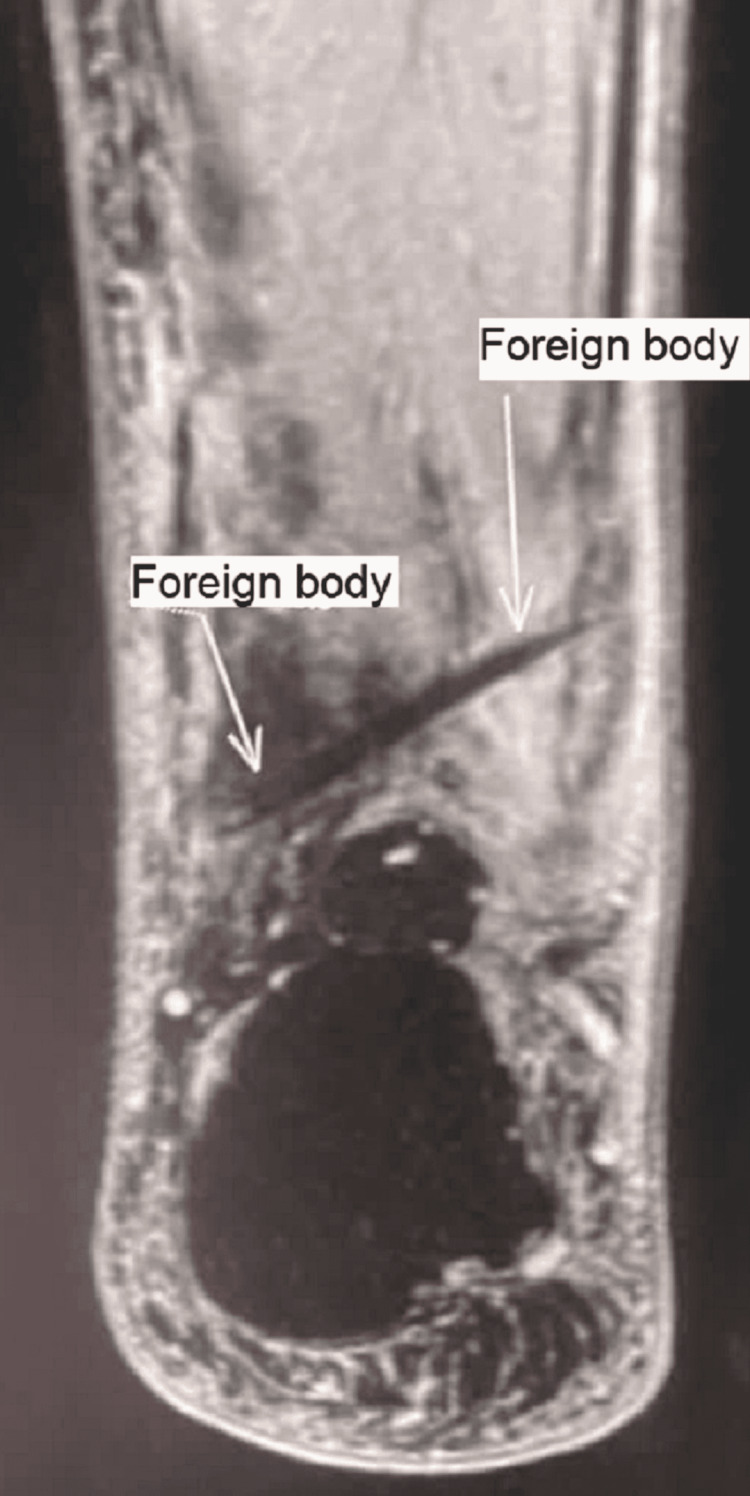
MRI in T2 sequence showing a foreign body marked by the arrows, hypointense, not compromising vascular or neural structures compatible with a barb.
